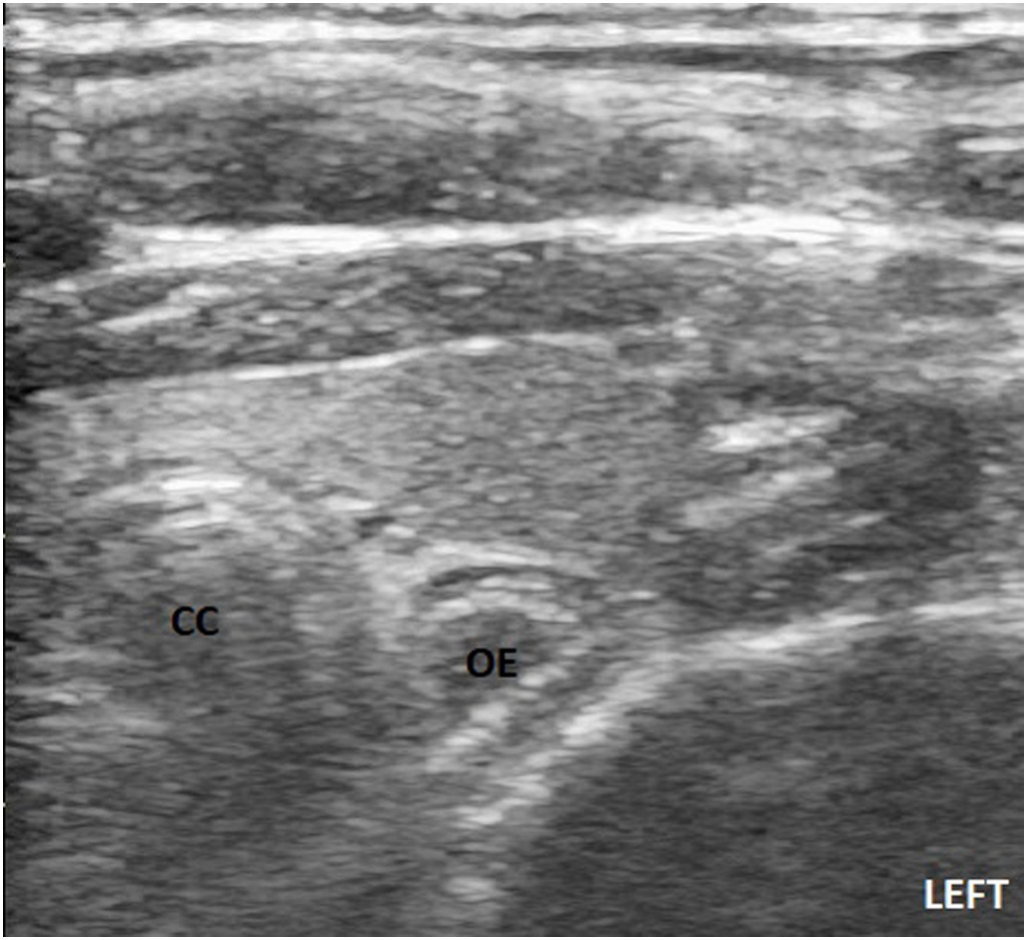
Ultrasound image of oesophagus before paralaryngeal pressure.
